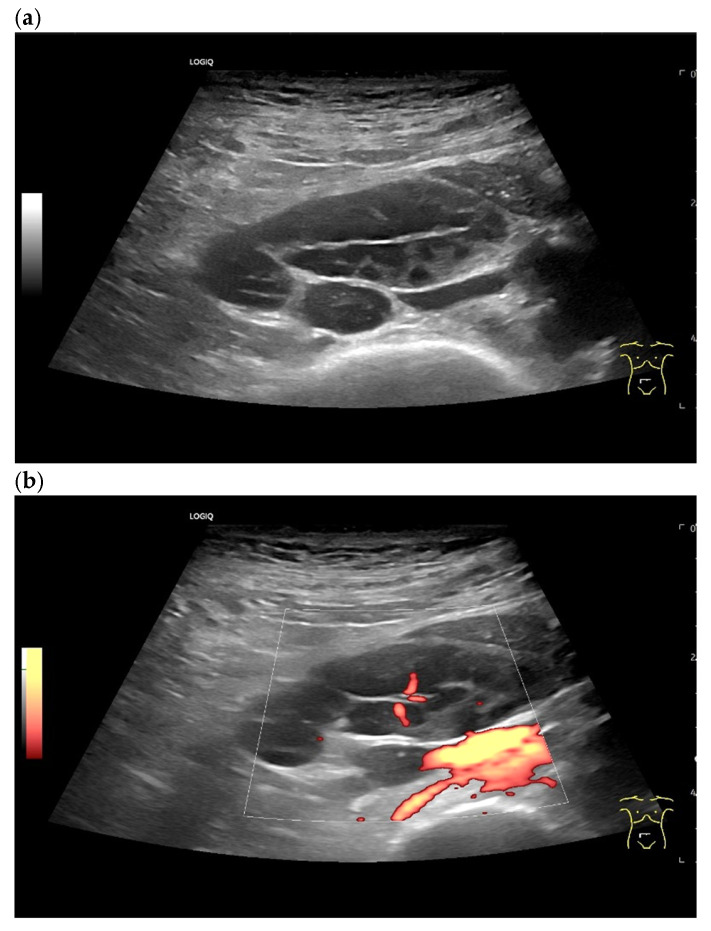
Chronic lymphocytic leukemia with watch-and-wait management. An enlarged paracaval LN measuring 30 × 20 mm is characterized by a compressed, narrow LN hilum and multiple hypoechoic lesions in the cortex (a). No feeding vessel is visible on power Doppler, but there are some small central vascular branches (vascular pattern II) (b).
